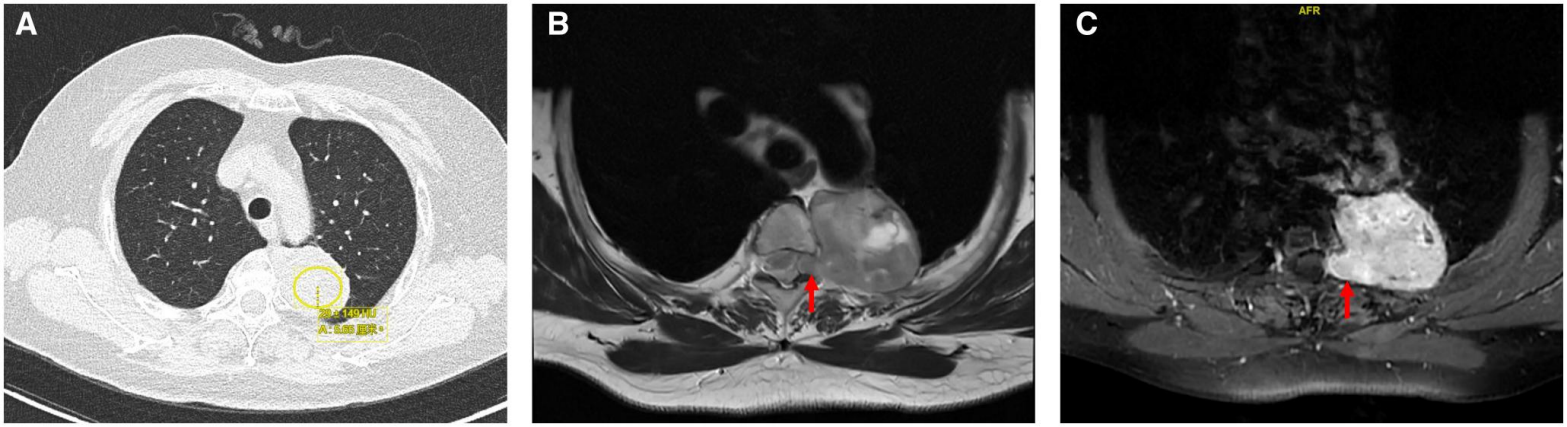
Preoperative Chest CT (A) Reveals a Soft Tissue Mass in the Posterior Upper Mediastinum, Adjacent to the Left Side of the T3 Vertebral Body, Measuring 5.5 × 3.9 cm with a CT Value of 29 ± 149 HU. Enhanced MRI (T1WI B and T2WI C) Demonstrates a Nodular Protrusion (Red Arrow) Extending from the Left T3/4 Intervertebral Foramen, Leading to Foraminal Stenosis and Thickening with Enhancement of the Adjacent Left Pleura
